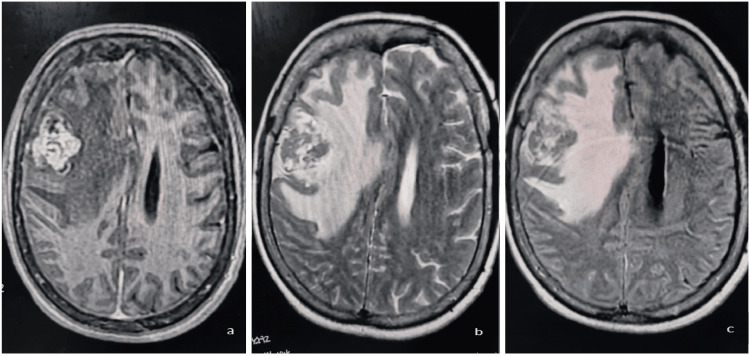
Brain MRI showing heterogeneous tumor with central necrosis, peritumoral edema, and leftward midline shift in right frontal lobe. (a) T1W sequence showing a hypointense lesion with heterogeneous internal areas. (b) T2W sequence demonstrating a heterogeneous hyperintense mass. (c) FLAIR sequence showing bright heterogeneous signal. T1W: T1-weighted; T2W: T2-weighted; FLAIR: fluid-attenuated inversion recovery
